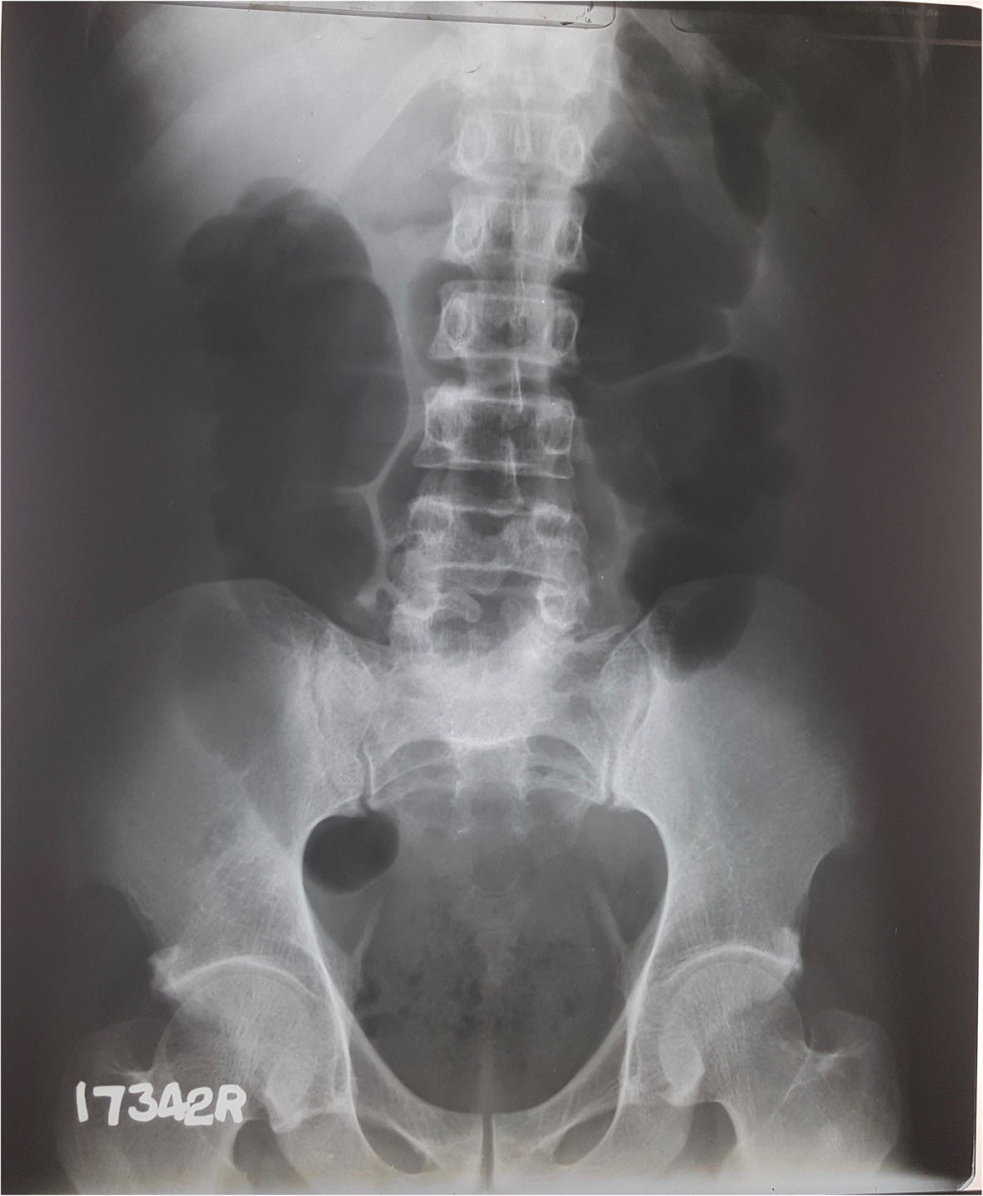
X-ray abdomen supine showing dilated ascending and descending colon with absent rectal air
